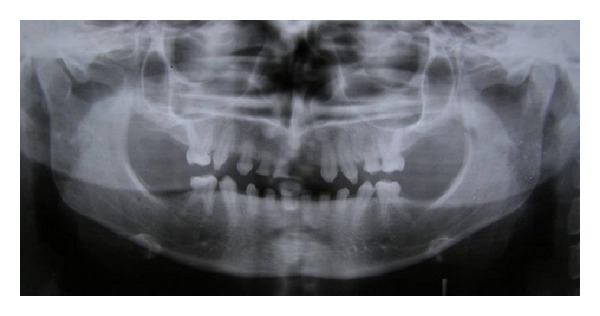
Preoperative radiograph showing overretained deciduous teeth and a few permanent teeth.
